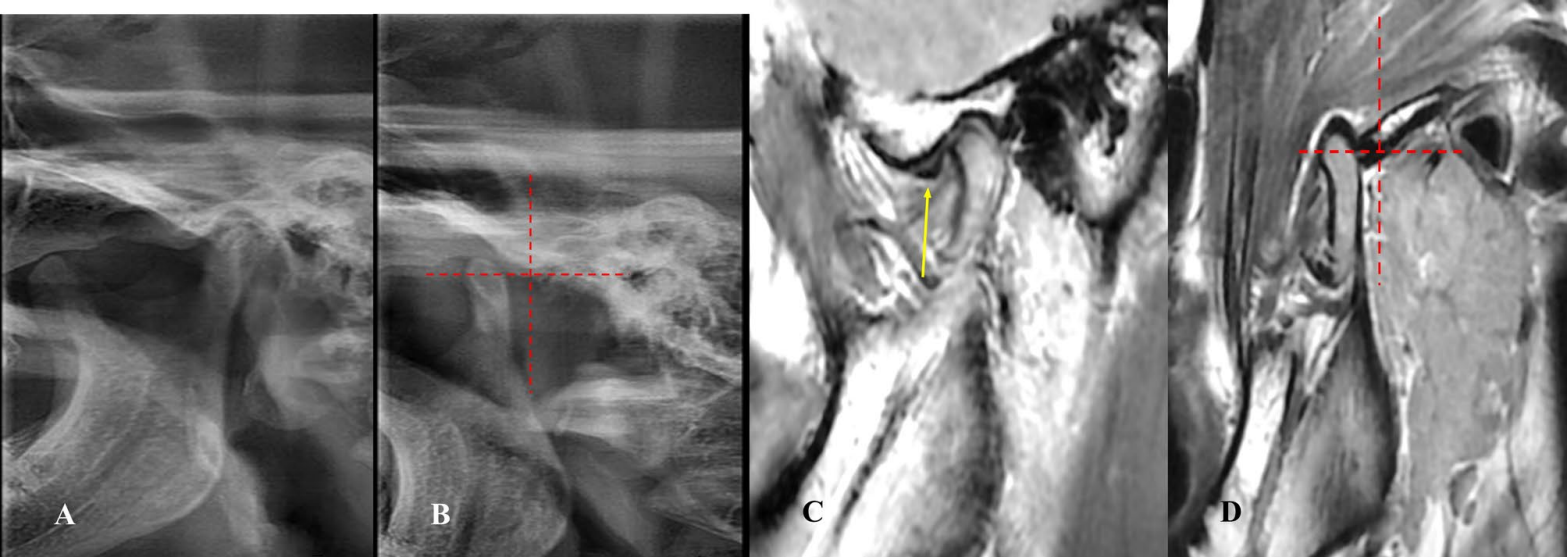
Lateral TMJ view represents stage II-B in closed (A) mouth position and in open (B) mouth position, showing the condyle located anterior to the eminence but at a superior level to the highest point of the eminence. Oblique sagittal T1-weighted images represent Stage II-B, showing anterior disk displacement (yellow arrow) in closed (C) mouth position with recapture of the articular disk (yellow arrow) during open (D) mouth position. The disk condyle complex positioned high up in front of the base of the eminence.
